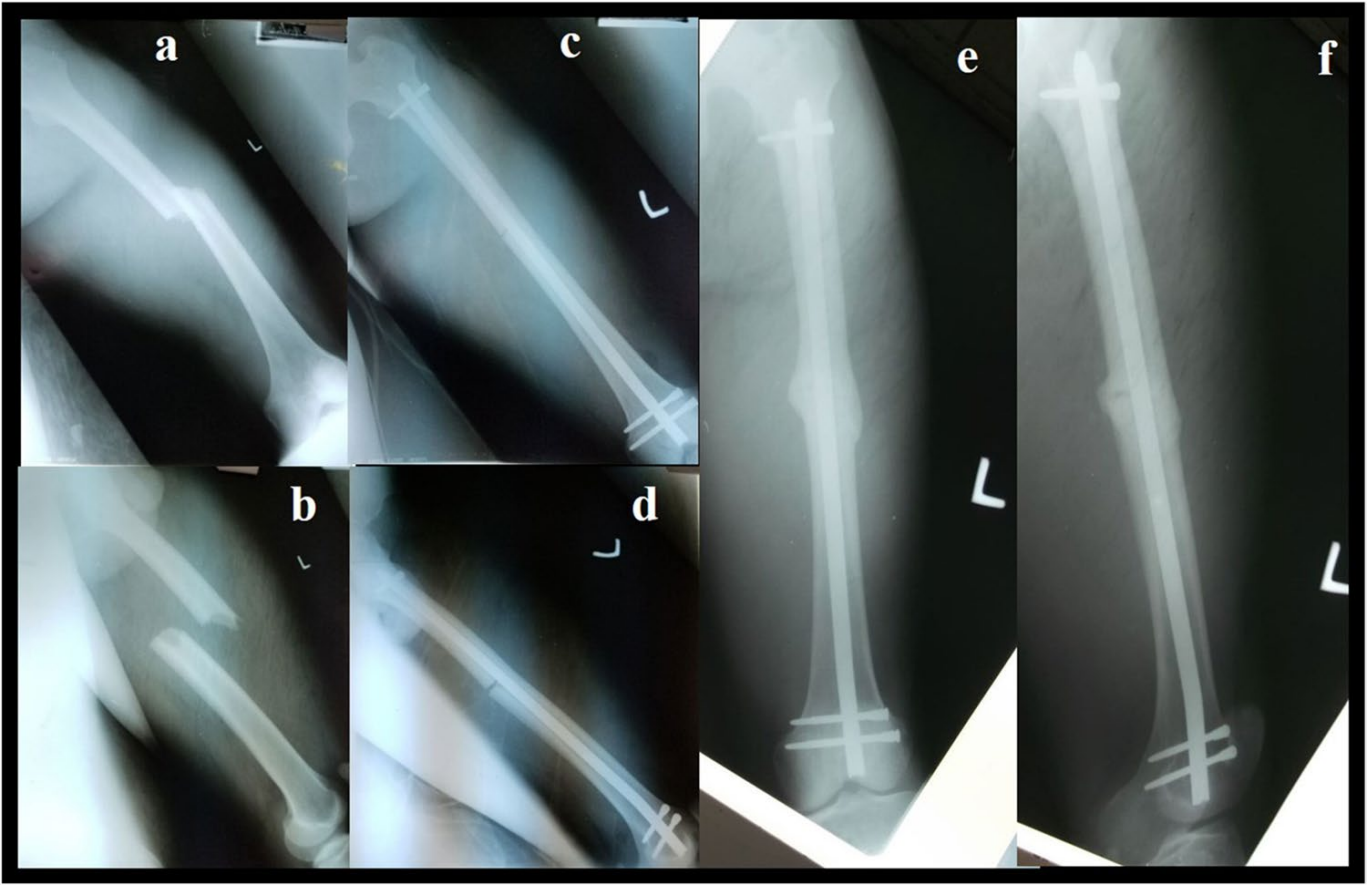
40 year old male patient with femoral midshaft fractures treated using SSN®, (a, b) preoperative radiographs, (c, d) postoperative radiographs, (e, f) consolidation at the follow up at 12 months
